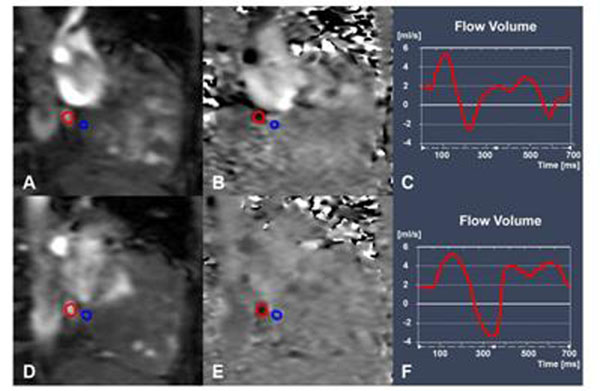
ROI Placement and Flow-Time-Curves. Phase contract MRI images of the CSF for a patient with scleroderma-associated PAH (mPAP 49 mmHg) during rest (top row: A, B) and adenosine-induced stress (bottom row: D, E). The red ROI is drawn around the coronary sinus, the blue ROI is placed in adjacent myocardium to correct for through-plane motion. The flow-time curves (C, F) demonstrate that net CSF increased only slightly from rest (0.86 ml/min/g) to stress (1.32 ml/min/g), resulting in a CFR of 1.53. Correspondingly, there is only little change in the diameter of and flow signal within the coronary sinus.
